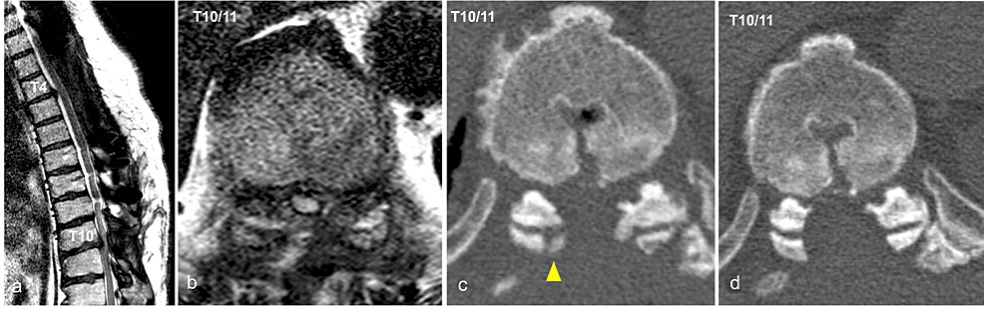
Images before revision surgery Sagittal (a) and axial (b) T2-weighted MRI images show severe spinal cord compression at T10/11. Axial CT before revision surgery (c) shows a recurrence of OLF at T10/11 (yellow arrowhead) compared to axial CT immediately after the initial surgery (d). MRI: magnetic resonance imaging, CT: computed tomography, OLF: ossification of the ligamentum flavum
